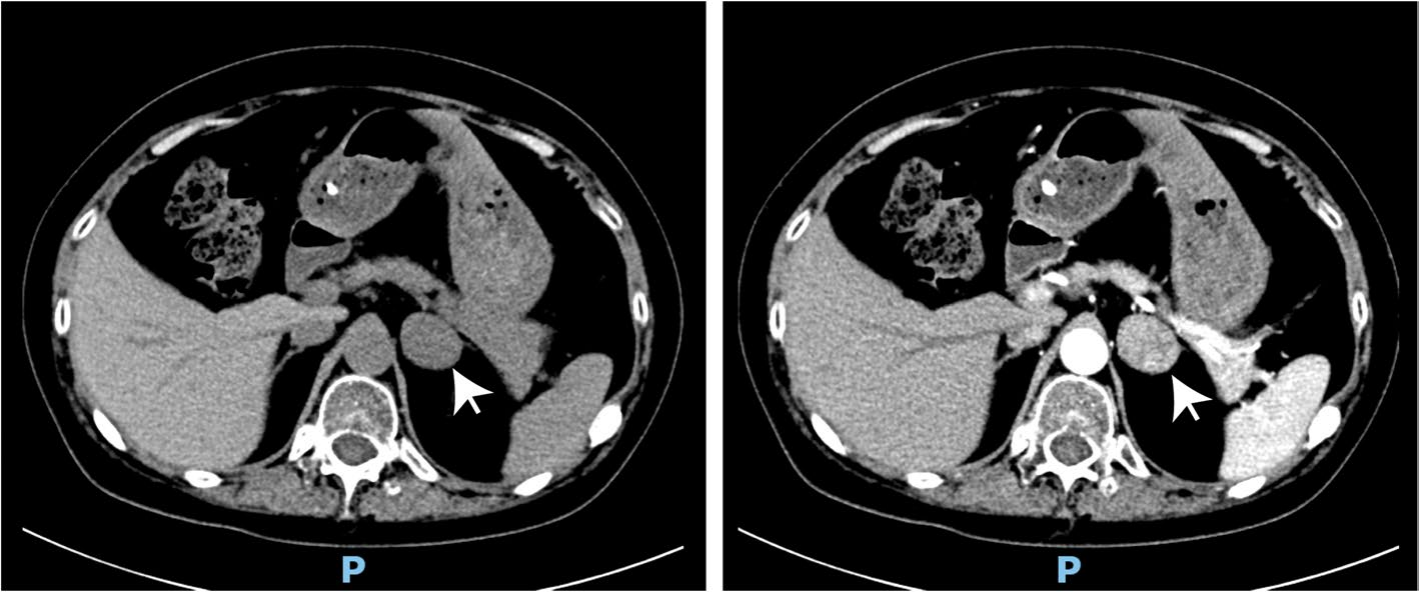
Adrenal gland CT showed a mass on left adrenal gland
